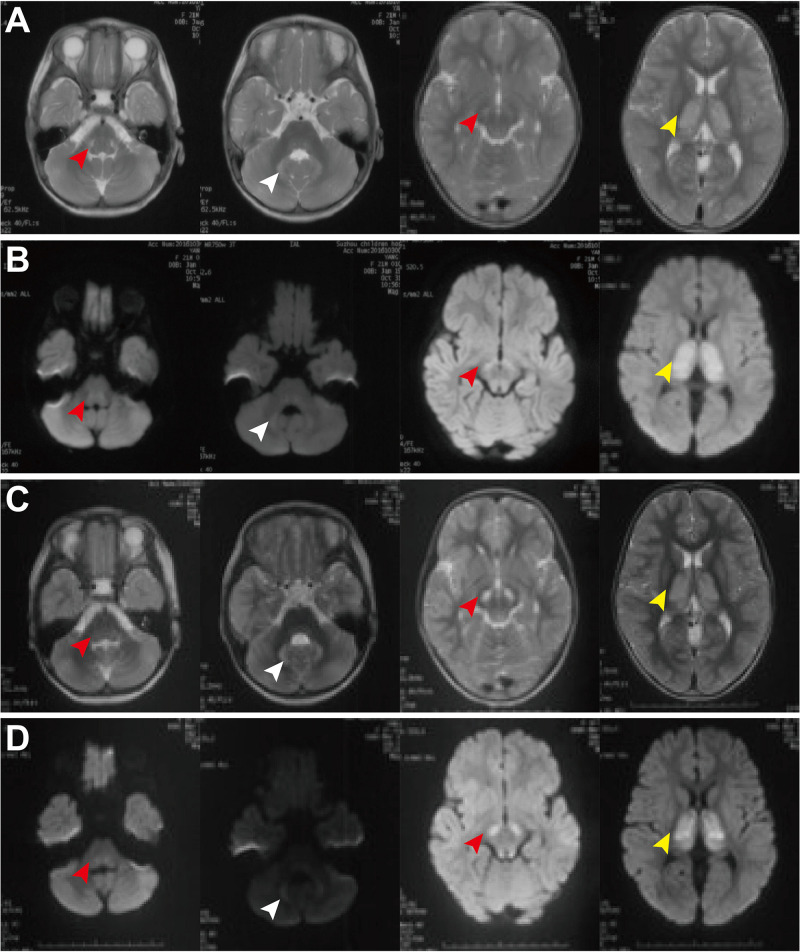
Brain MRI of patient 3 at the ages of 1 year and 9 months old (A,B) and 3 years and 8 month old (C,D). MRI demonstrated bilateral lesions in the brain stem (red arrowheads), thalamus (white arrowheads), and dentate body of cerebellum (yellow arrowheads), with diffusion limitation in diffusion weighted image. There is no significant improvement during the follow-up period.
